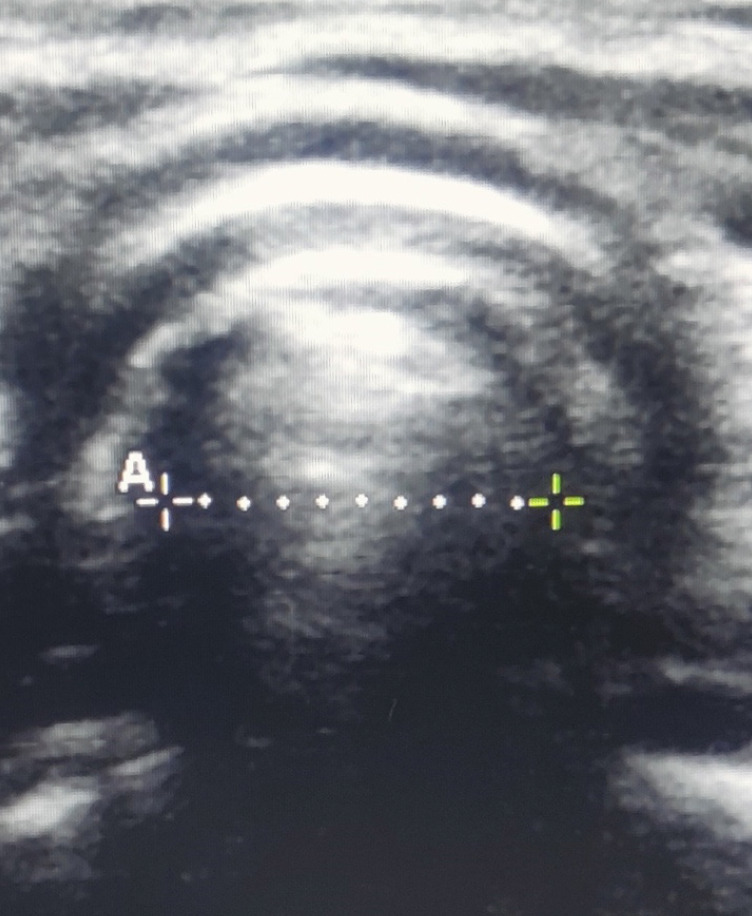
USG view of cricoid arch and air-column. Cricoid cartilage is a round hypoechoic structure with hyperechoic edges. The air column (dotted line) appeared hyperechoic and created a posterior acoustic shadow. The mucosa-air interface, a hypoechoic edge, was easily recognized. The dotted line represents the measured air-column width. USG, ultrasonography.
